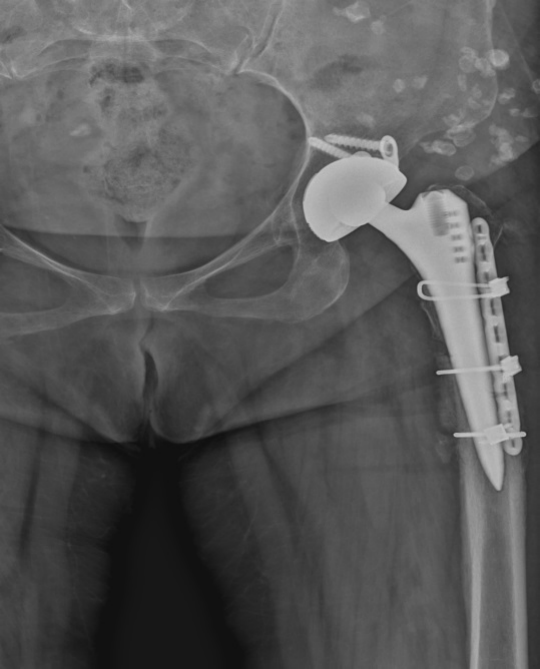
X-ray radiograph of patient who underwent osteotomy fixation with plate and cable after union.
